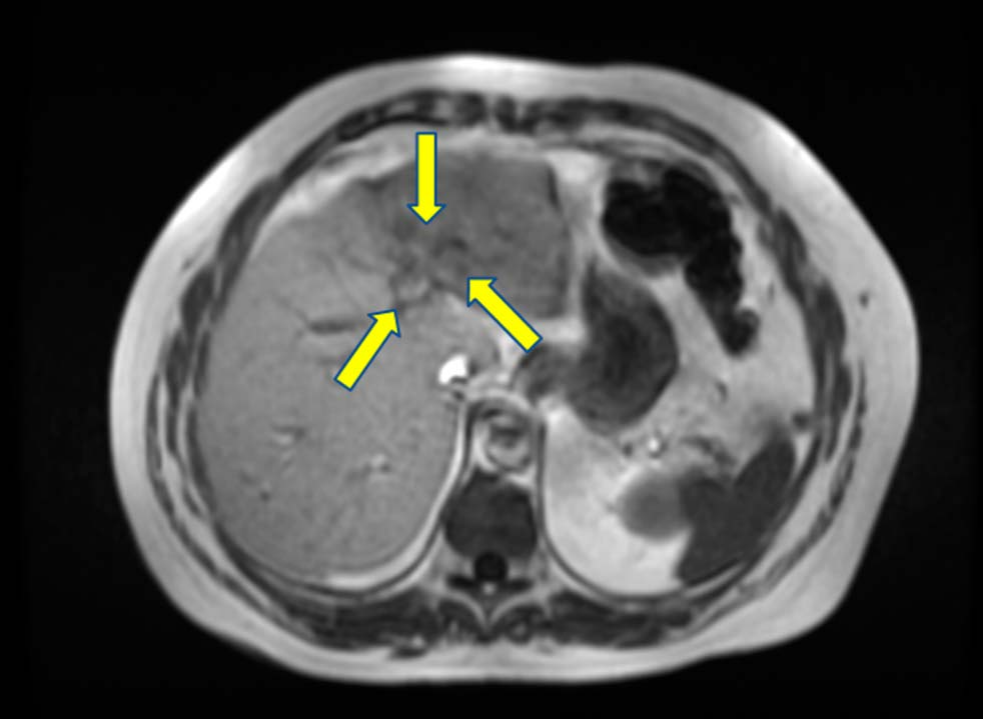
T1-weighted imaging of the liver mass.
